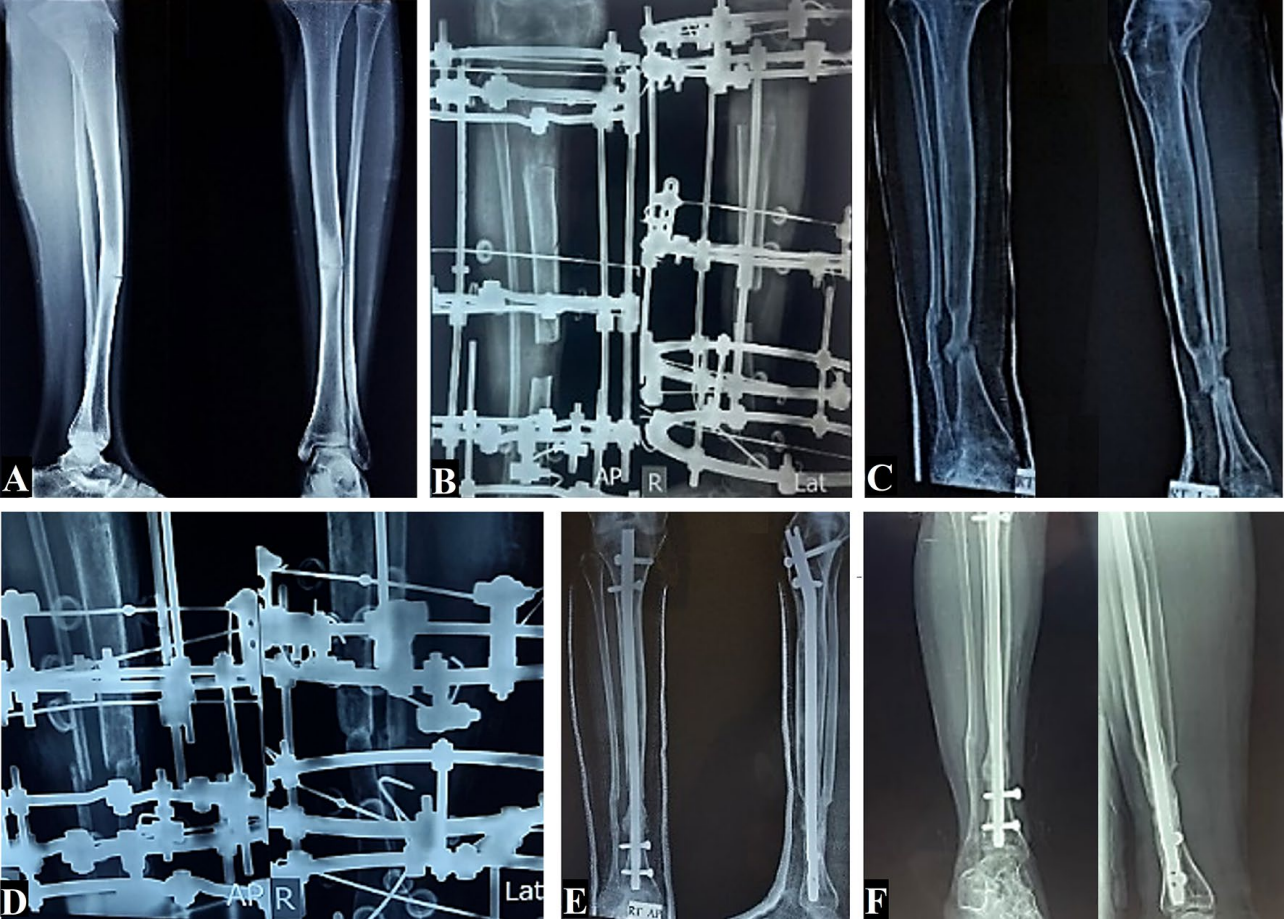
A 14.5-year-old female with congenital segmental tibial dysplasia without pseudarthrosis. A Preoperative AP and lateral X-rays. B Postoperative AP and lateral X-rays of Ilizarov segment transport after resection of the diseased segment. C Recurrence of sclerosis and narrow medulla with established pseudarthrosis 4 months post fixator removal. D Revision using another Ilizarov frame after limited debridement. E Maintenance of union by interlocking tibial nail. F AP and lateral X-rays showing complete union at 5.5 years’ follow-up
